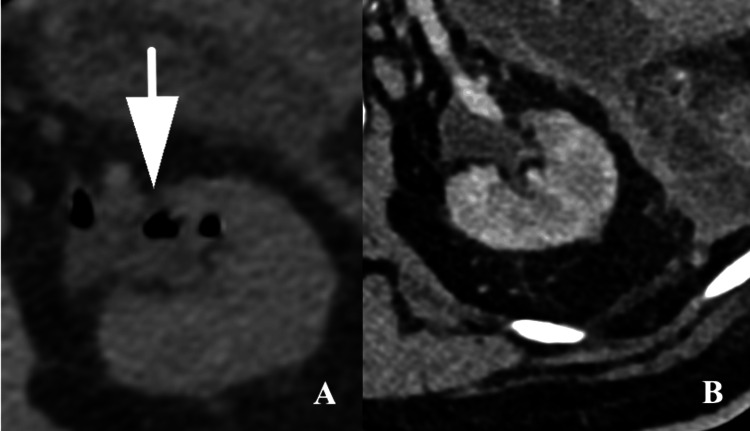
A) Axial non-contrast CT of the left kidney showing gas within the renal collecting system on the initial imaging, and B) Axial contrast enhanced CT of the left kidney five days later showing near complete resolution of this gas.
